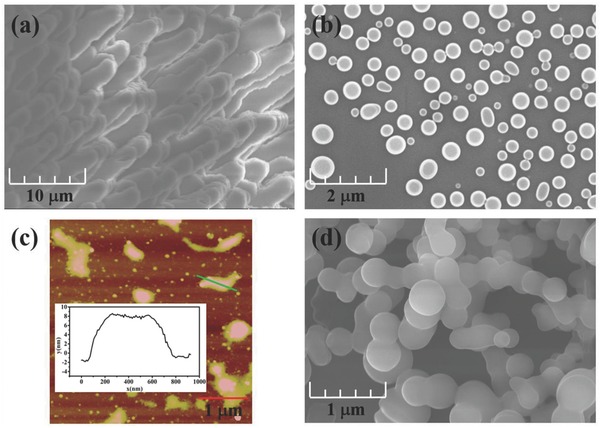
a) SEM image of cross‐sectional morphology of MCDF‐1 crystal sample. b) SEM image of MCDF‐1 disk‐like micro/nanosheets obtained by ultrasonic treatment in a deionized water solvent. c) AFM image of MCDF‐1 nanosheets obtained by ultrasonic treatment. d) SEM image of MCDF‐1 elliptical‐like micro/nanosheets obtained by ultrasonic and grinding treatment.
